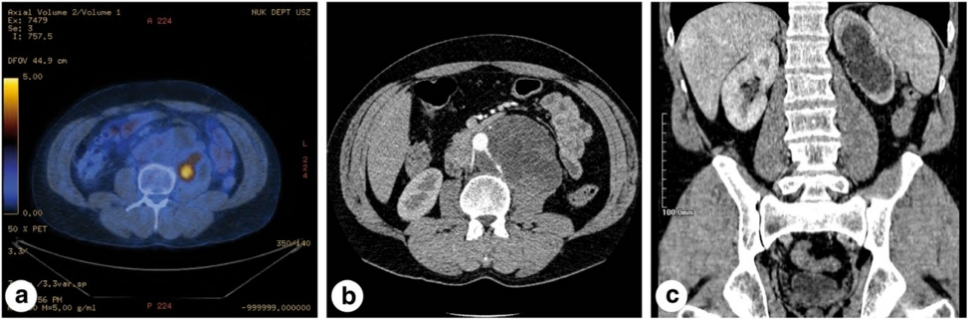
Pre-operative positron emission tomography showing a large retroperitoneal mass with a centrally located metabolic activity of 2.4 cm in diameter (a). Computed tomography (CT) with the large retroperitoneal mass and involvement of the renal vessels (b). A kidney tumour was detectable neither in the right nor in the left hydronephrotic kidney (c).
